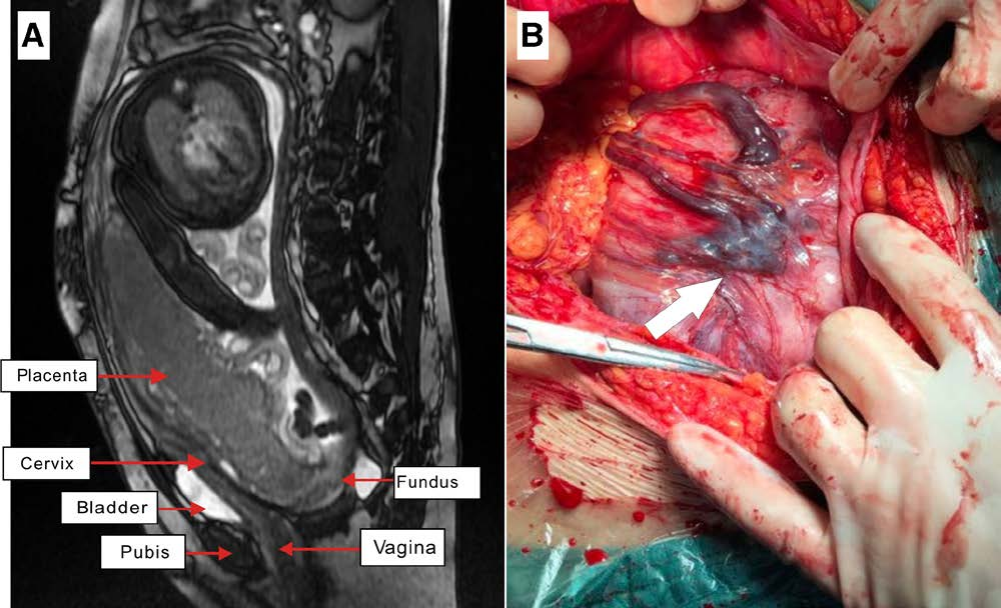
MRI image and intraoperative photograph of the second patient. (A) The image demonstrates extreme retroflexion of the uterus. (B) The photograph shows the upper edge of uterus traversed by a large number of dilated blood vessels. MRI = magnetic resonance imaging.
